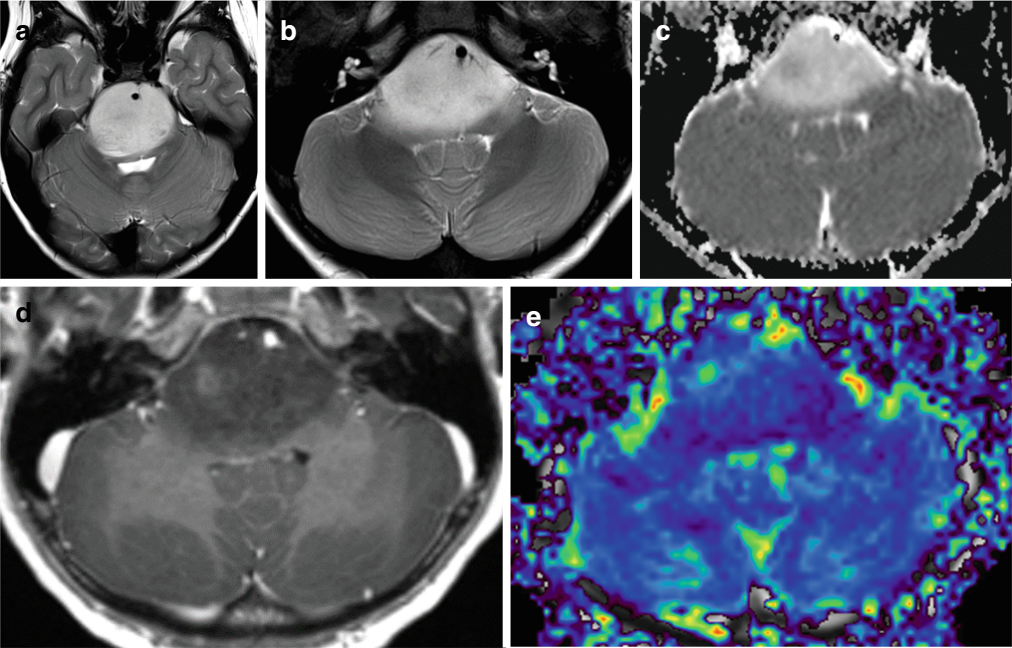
a–e Diffuse midline glioma with alteration of histone H3 at position 27 (H3K27-altered) (female, age: 7 years) with epicenter in the pons showing exophytic growth and encasement of the basilar artery (a, b). Focally low values for the apparent diffusion coefficient (ADC values) (c) indicate high cellularity, only focal contrast enhancement is present (d). High values for the relative cerebral blood volume (rCBV values) (e) indicate a pronounced vascularization. a–b T2-weighted images, c ADC map, d T1-weighted image after i.v. application of contrast agent, e colored rCBV map. Biopsy and molecular biological analysis revealed a H3K27M alteration. For diffuse gliomas located in the midline, an alteration of histone 3 at position 27 due to a mutation or due to other alterations such as enhancer of zeste homologs inhibitory protein-overexpression (EZHIP overexpression) or EGFR-mutation is diagnostic for a diffuse midline glioma, H3K27-altered
